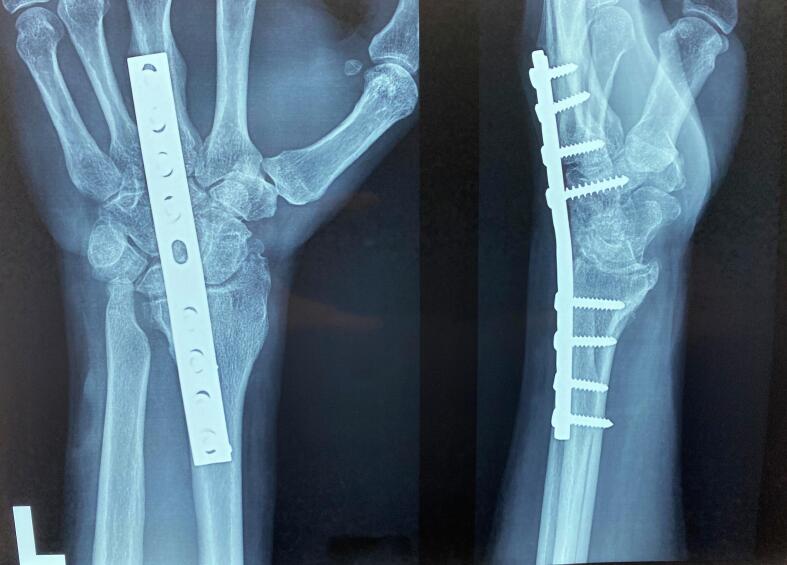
Antroposterior and lateral plain radiographs of the left wrist demonstrates six months post arthrodesis surgery with plate and screws. Bower's hemiresection-interposition arthroplasty was performed for distal radio-ulnar arthrosis.
